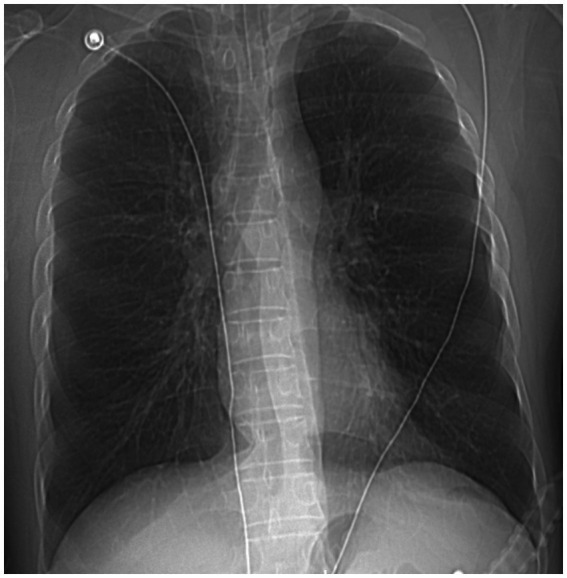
A chest radiograph shows no obvious abnormalities in both lungs.
